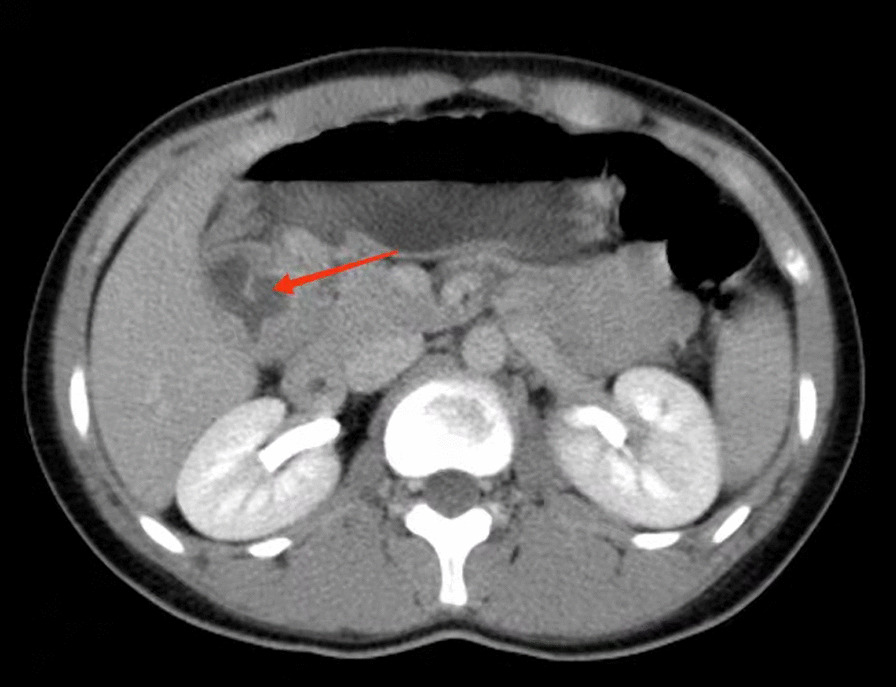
CT scan showed a thick-walled cystic lesion with an enhanced outline of the cystic wall in the antrum of stomach, suggestive of duplication cyst (arrow)
